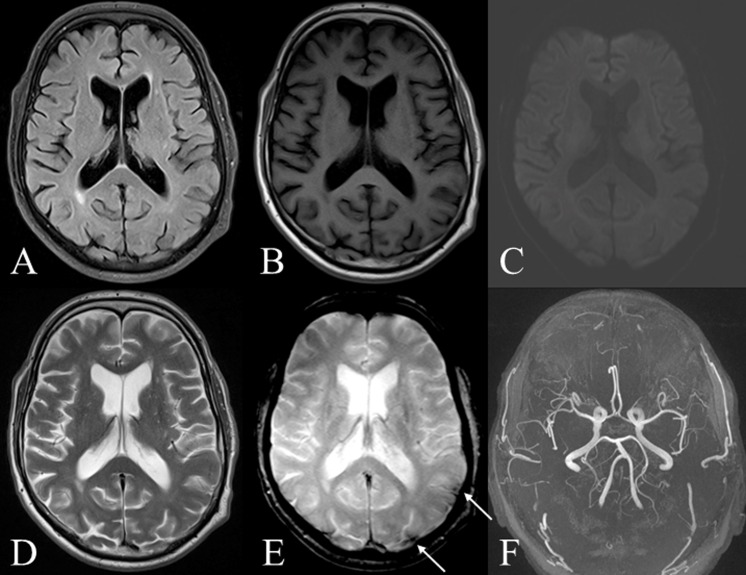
MRI follow-up eight years after nonperimesencephalic subarachnoid hemorrhage of a 73-year-old male patient (Table 1, #17). Post-hemorrhagic sulcal siderosis is indicated by arrows. *A-D* show no residuals after bleeding. *E* shows sulcal siderosis on the parietal and occipital left hemisphere. *F* depicts no aneurysm in TOF-angiography. Sequences: *A* = T2 TIRM weighted, *B* = T1-TIRM weighted, *C* = diffusion-weighted, *D* = T2 weighted, *E* = T2* weighted, *F* = time-of-flight-angiography.
